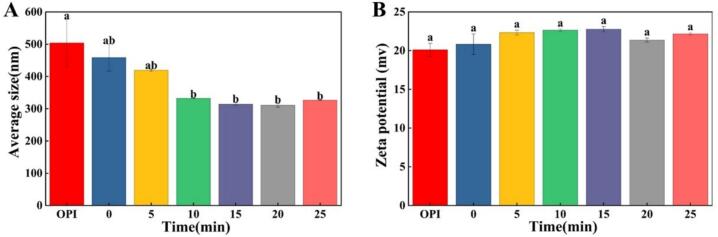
(A) Mean particle size and (B) zeta potential of OPF subjected to different ultrasonic treatment times (0, 5, 10, 15, 20, 25 min) and untreated OPI. Different letters indicate significant differences (*P* < 0.05).
